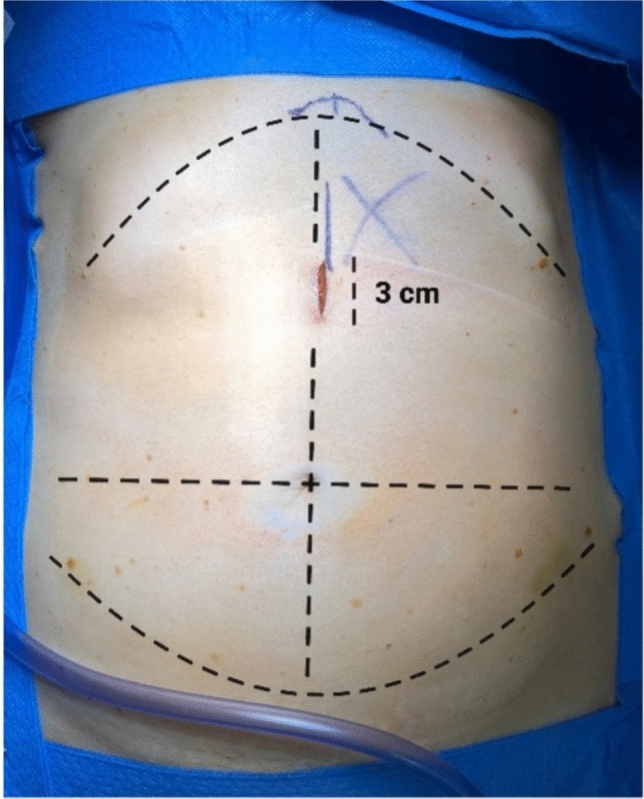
Access: A 3–4 cm supraumbilical midline incision is created. The precise location is determined according to the stomach’s position by reviewing the CT scan
